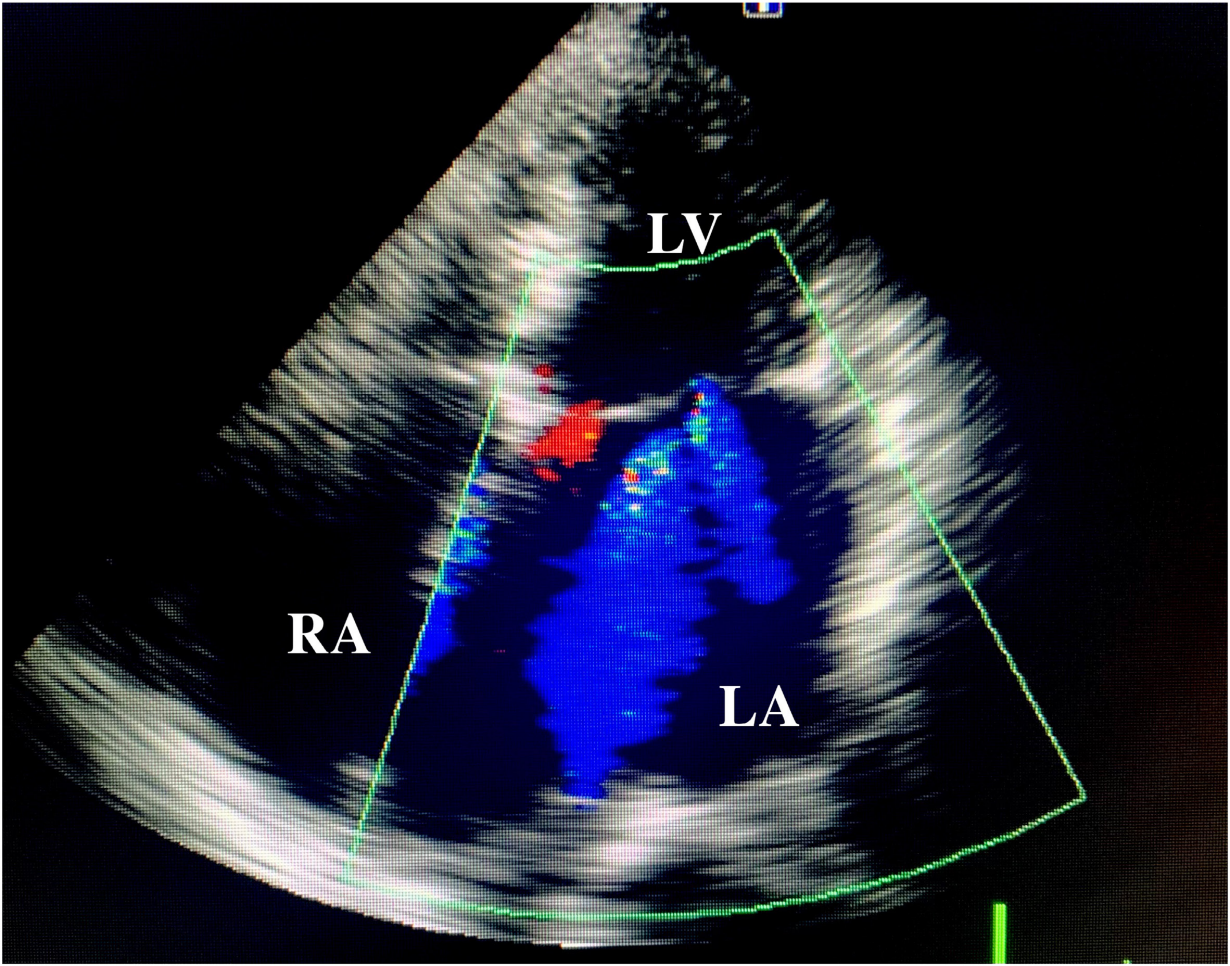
Transthoracic echocardiography imaging showing severe mitral valve regurgitation and severely dilated left atrium. LA, left atrium; LV, left ventricular; RA, right atrium.
